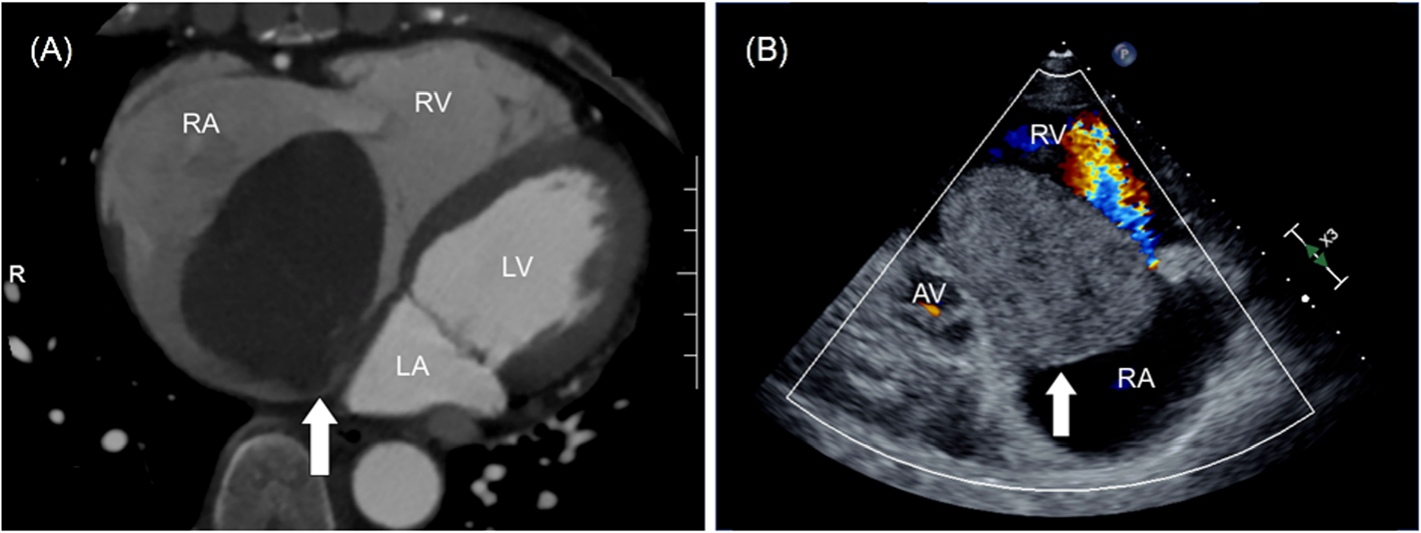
a CT showing a large mass in the right atrium (white arrow). b Preoperative TTE showing a 57 mm × 63 mm giant tumor obstructing the tricuspid valve inflow (white arrow). RA, right atrium; RV, right ventricle; LA, left atrium; LV, left ventricle; AV, aortic valve
